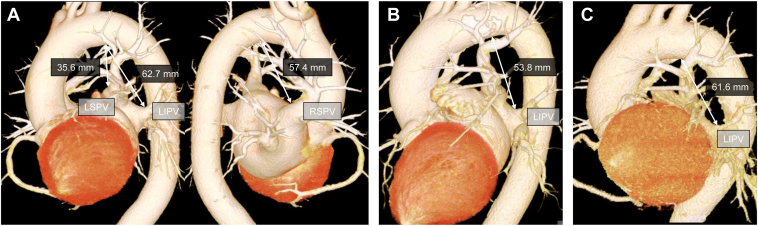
Anatomical distances from the PV ostia to the inferior border of the aortic arch, assessed by 3-dimensional CT. A: Case 1 image shows distances from the LSPV (35.6 mm), LIPV (62.7 mm), and RSPV (57.4 mm) ostia to the inferior border of the aortic arch. This border approximates the left recurrent laryngeal nerve path. B: Case 2 image shows the LIPV–aortic arch distance as 53.8 mm. C: Case 3 image shows the LIPV–aortic arch distance as 61.6 mm. CT = computed tomography; LIPV = left inferior pulmonary vein; LSPV = left superior pulmonary vein; PV = pulmonary vein; RSPV = right superior pulmonary vein.
